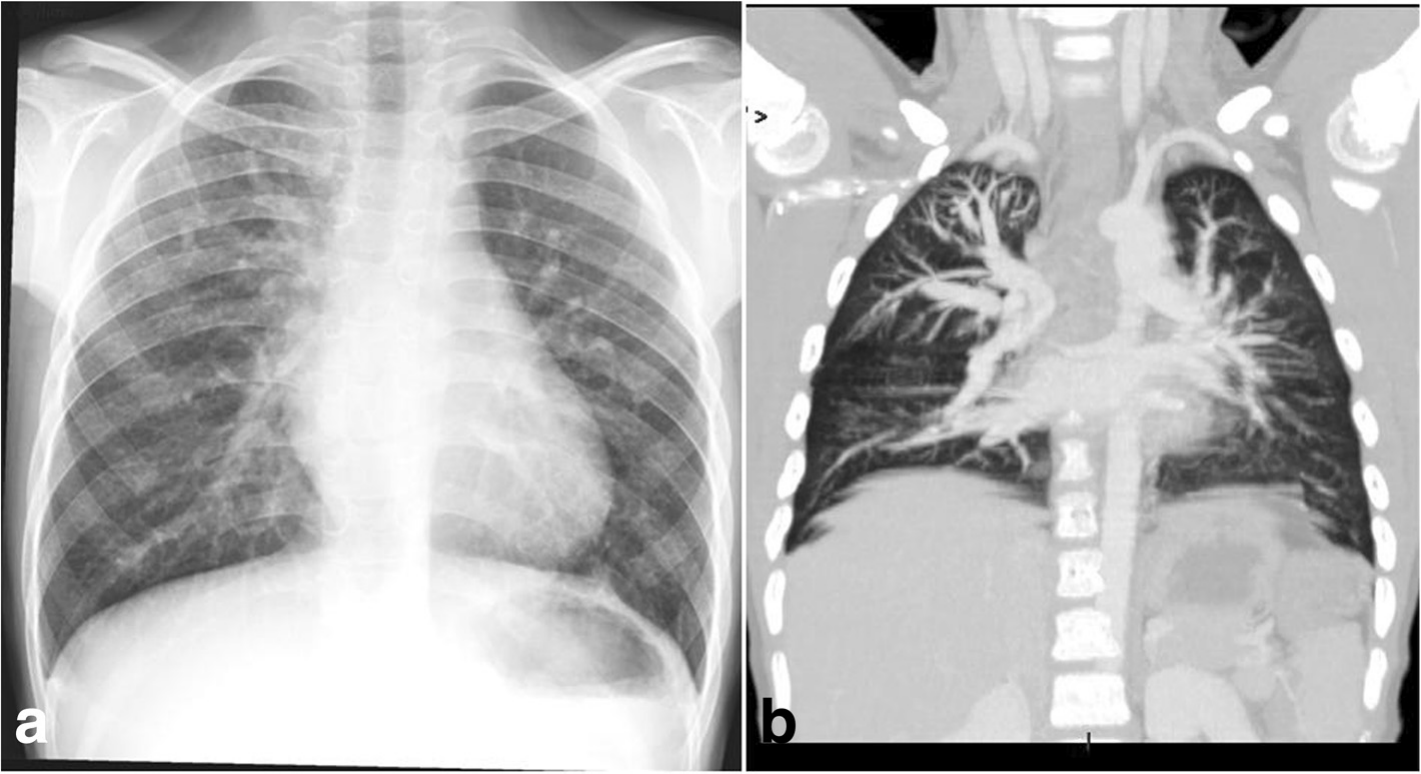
Pre-procedure chest radiogram (Panel a) and coronal multiplanar reconstruction (Panel b) of contrast-enhanced multidetector computed tomography showing increased pulmonary vascularity of the right lung mainly in the upper lobe
